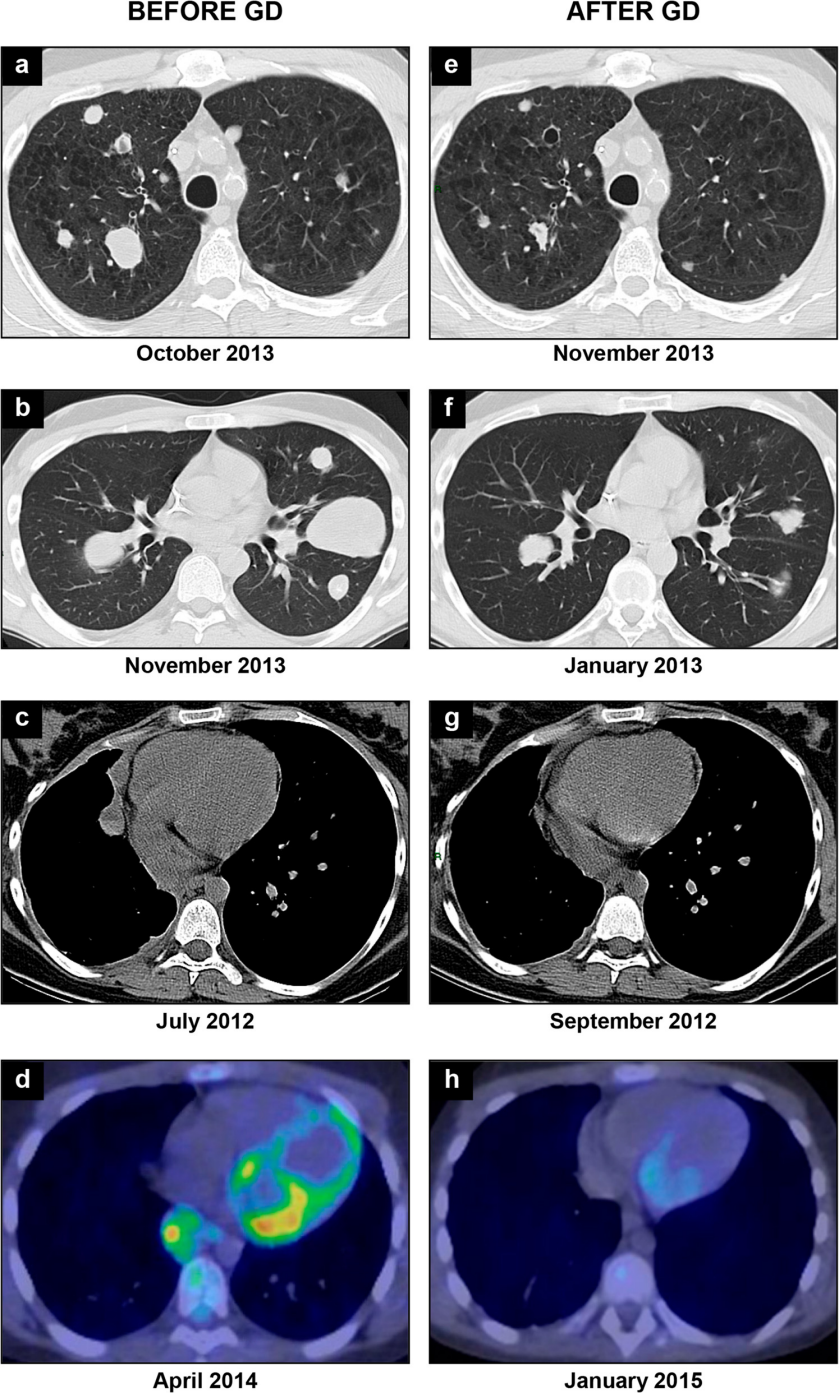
RECIST responses by chest CT scan in 3 patients with bilateral lung metastases, before gemcitabine and docetaxel (GD) treatment (a, b, c) and after 2 cycles of therapy (e, f, g); PET/CT imaging showing 18 F-FDG uptake in a patient with osteosarcoma lung metastastases, before GD treatment (d) and after 7 cycles of therapy (h)
